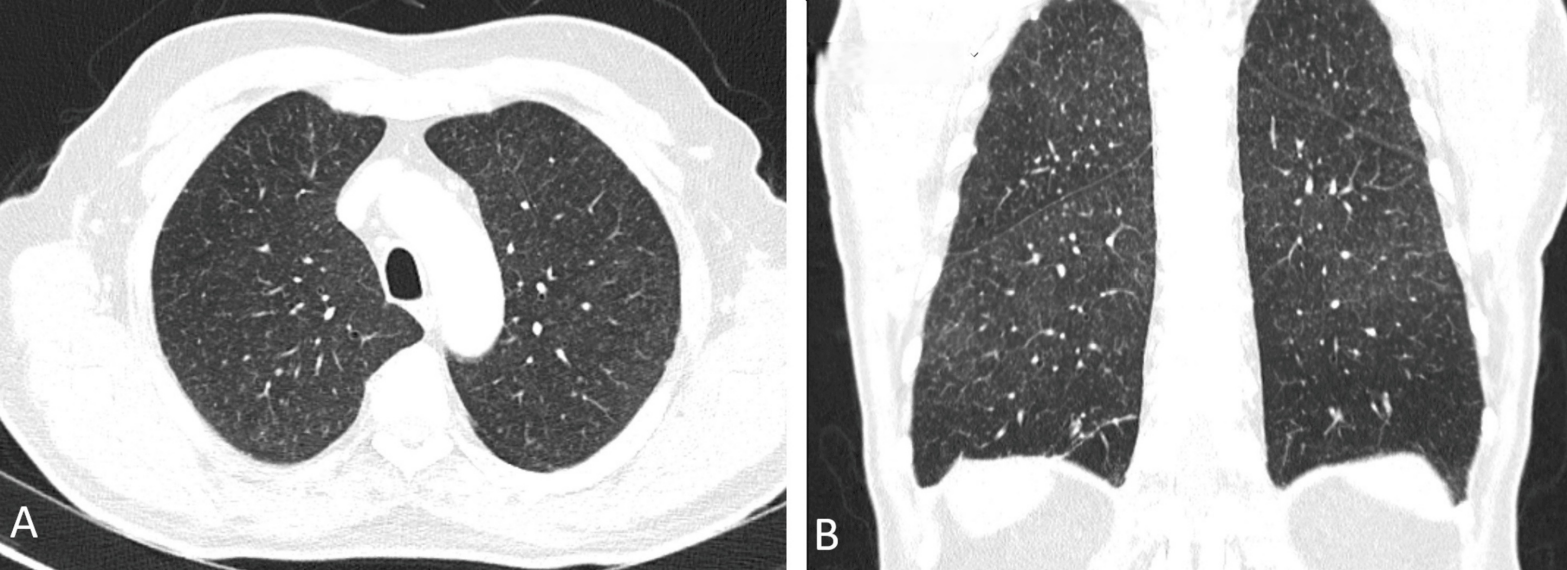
Computed tomography. Axial (A) and coronal (B) images showing expanded lungs with peribronchovascular interstitial thickening of diffuse micronodular appearance in both lungs, suggestive of a nonspecific inflammatory process of the small airways. No pulmonary consolidations are present.
